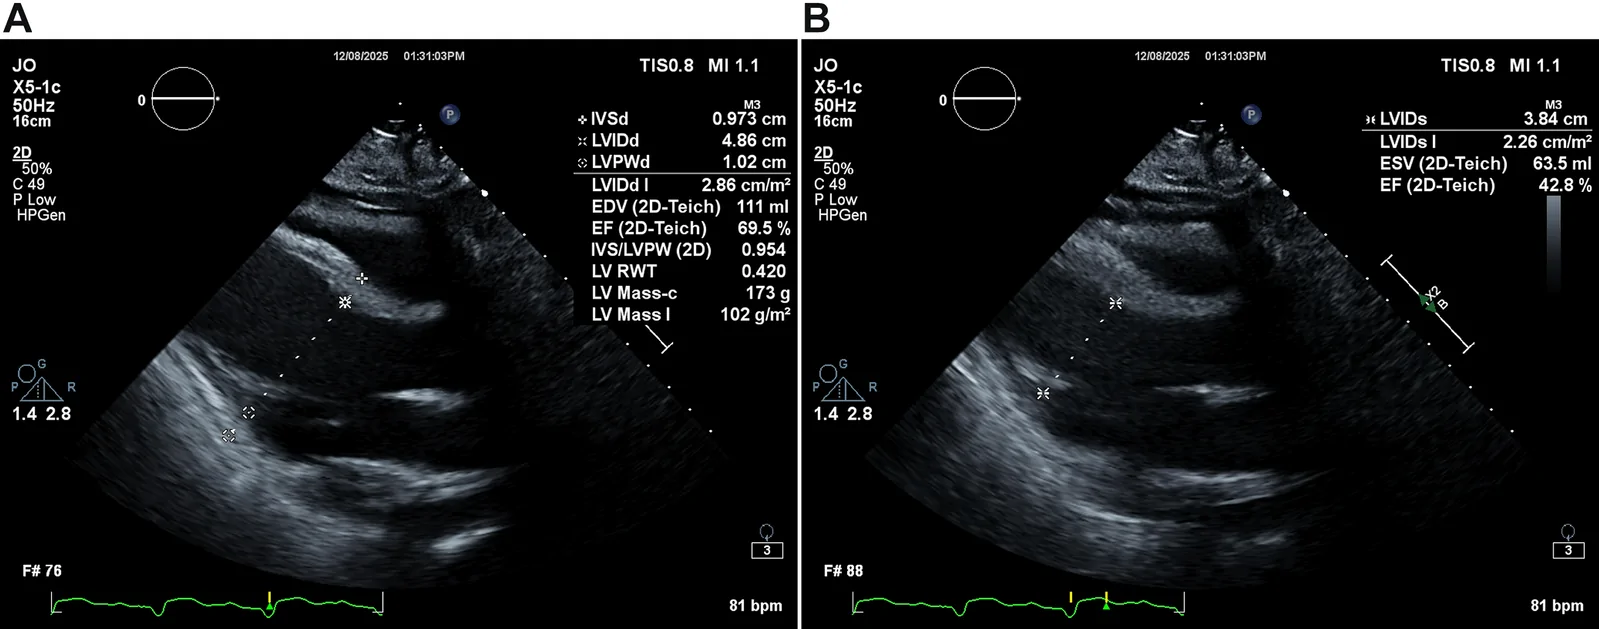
Parasternal Long-Axis View of the Transthoracic Echocardiogram Demonstrating Improvement of Left Ventricular Ejection Fraction of 42% After Initiation of Finerenone (A) End-diastole with left ventricular internal dimension at end-diastole (LVIDd) of 4.9 cm and (B) End-systole with left ventricular internal dimension at end-systole (LVIDs) of 3.8 cm. EDV = end-diastolic volume; ESV = end-systolic volume; IVSd = interventricular septum thickness at end-diastole; LVPWd = left ventricular posterior wall thickness at end-diastole; LV = left ventricular; RWT = relative wall thickness.
